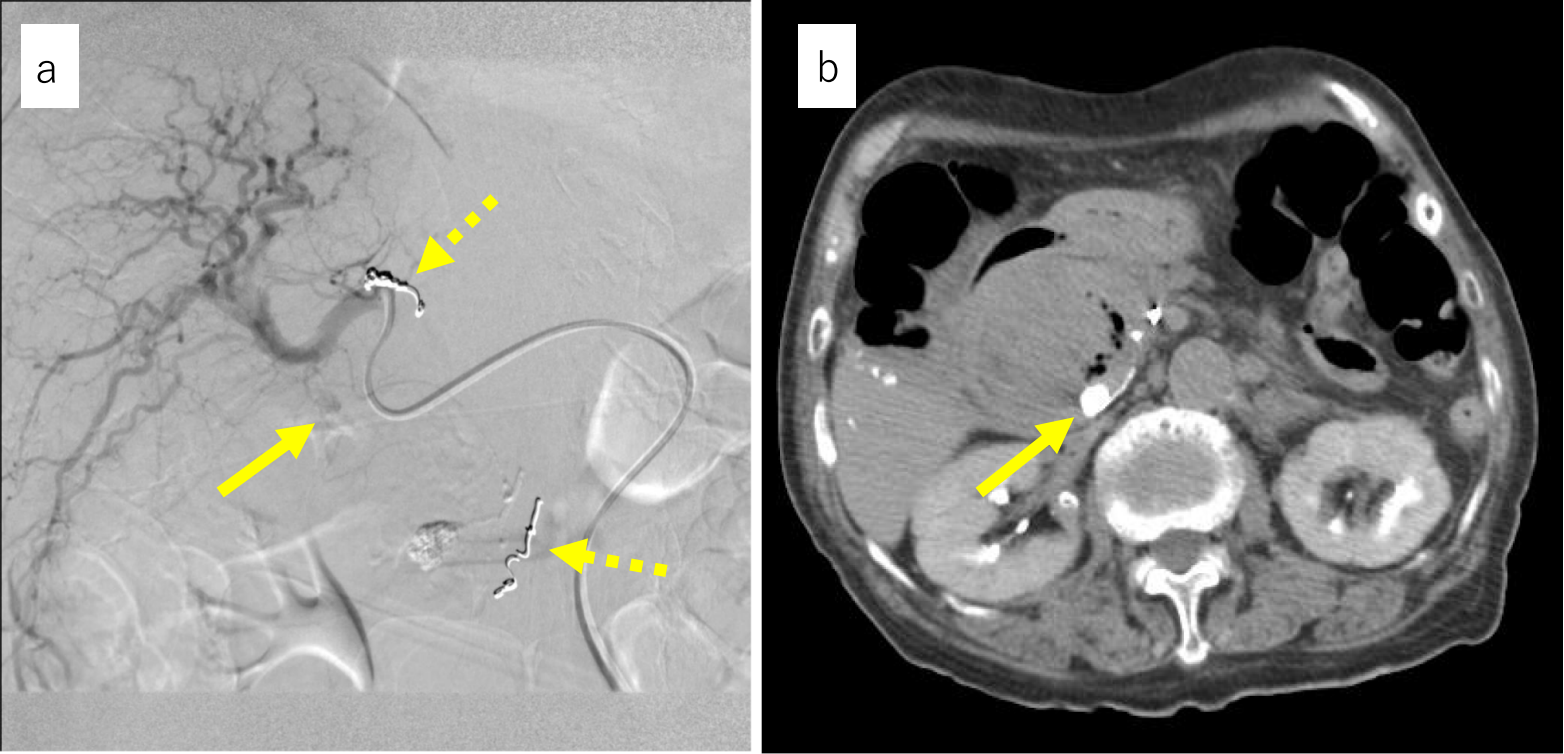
Findings from angiography performed in our hospital. a Contrast image of the proper hepatic artery is shown. The inferior pancreaticoduodenal artery and branch of the proper hepatic artery were coiled at a previous hospital (dotted arrow). A solid arrow shows extravasation. The culprit vessel was suspected to be the PBP, which is a branch of the proper hepatic artery. b Transverse CT image after embolization with lipiodol and n-butyl-2-cyanoacrylate is shown. An arrow shows embolized pseudoaneurysm
